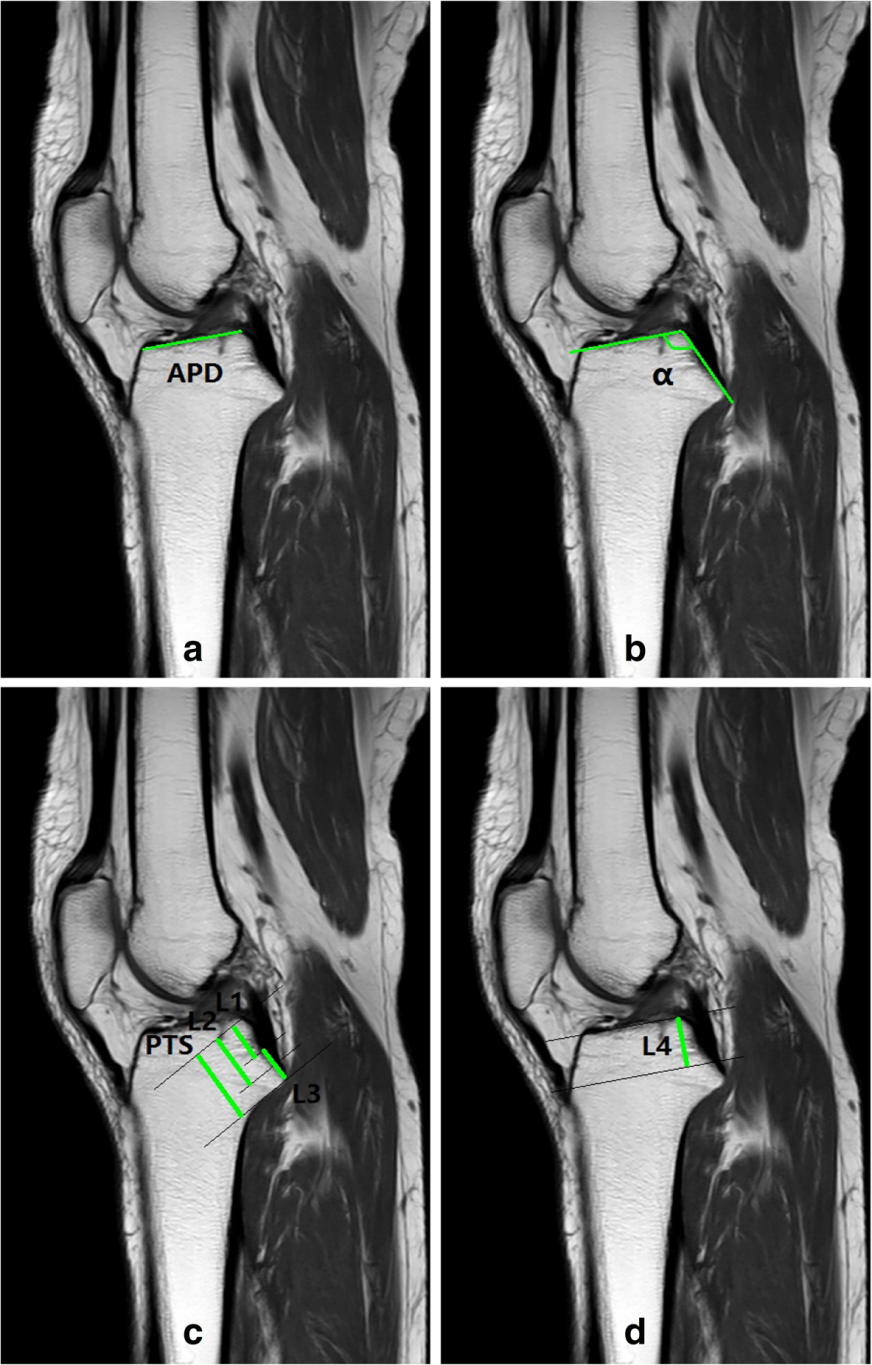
The relevant measurements of the PCL tibial attachment. a MRI showing measurement of the APD of the tibial plateau; b MRI showing measurement of the angle (α) between the tibial plateau and PTS (posterior tibial ‘shelf’); c MRI showing measurement of the distance from the proximal (L1) and central (L2) positions of the tibial PCL attachment to the tibial plateau, the width (L3) of the attachment, and the length of the PTS; d MRI showing measurement of the vertical dimension (L4) of the PCL central attachment site from the tibial plateau
